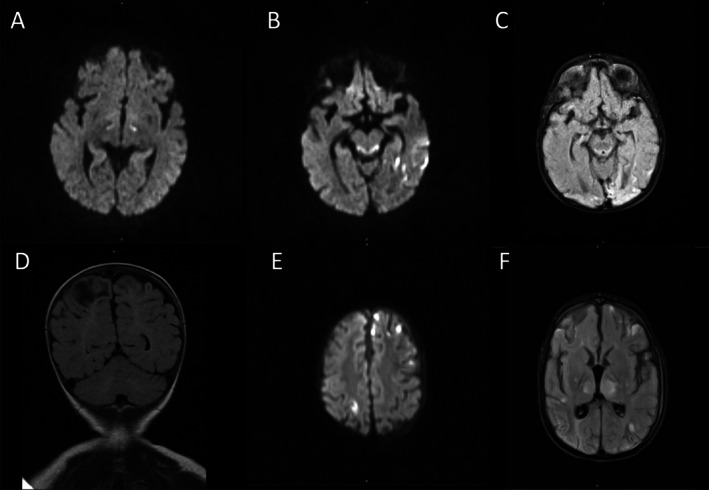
Brain imaging of the probands. (A–C) Brain MRI of Patient 1: (A) Brain MRI at the age of 21 months of age demonstrated reduced diffusivity in bilateral thalami on diffusion weighted imaging (B) At the age of 25 months, brain MRI showed reduced diffusivity in bilateral dorsal midbrain and left dorsal cerebral cortices on diffusion weighted imaging. (C) At 33 months of age, brain MRI showed gliosis in brainstem and left dorsal cerebral cortices as well as evolving ischemic injury in right dorsal cerebral cortices on T2/FLAIR imaging. (D–F) Brain MRI of Patient 2: (D) Brain MRI at 3 months of age demonstrated encephalomalacia in right greater than left parietal cortices on T2/FLAIR imaging. (E–F) Brain MRI at 3 years of age showed multifocal distribution of decreased diffusivity on diffusion weighted imaging (E) and hyperintensity on T2/FLAIR imaging (F).
